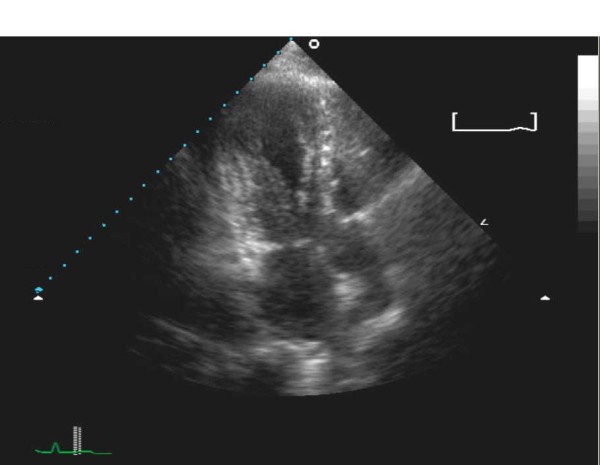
Echocardiography findings of apical hypokinesia in a two-chamber view.
